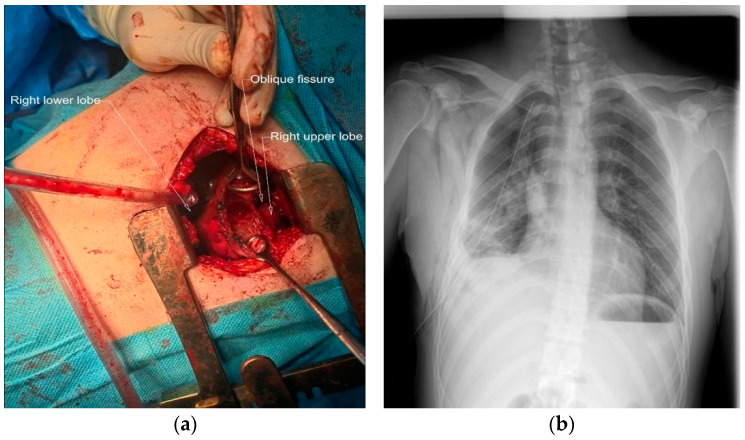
Surgical treatment of the large emphysematous bulla. (a) Right antero-lateral thoracotomy: incision of the emphysematous bulla in the right lower lobe and suturing of the fistula at this level. (b) Chest-X-ray first day post-surgery, showing a medium-intensity opacity occupying the inferior right pleural cavity.
